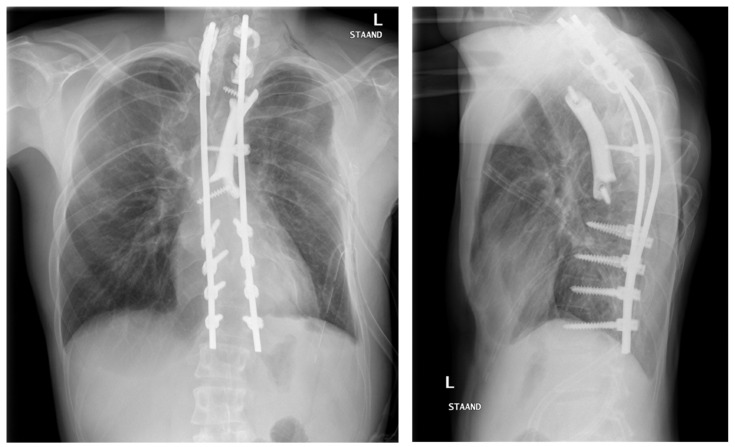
Case 3: Anterior-posterior (left) and sagittal (right) radiographs postoperation.
